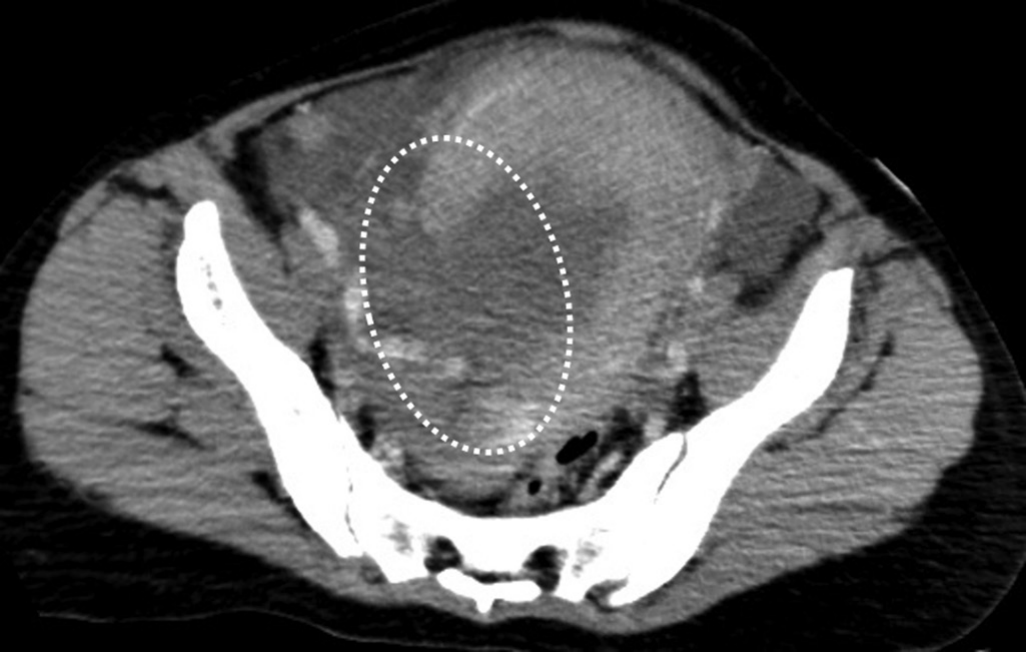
Contrast‐enhanced computed tomography axial image at the time of arrival. This image shows separation of the uterine wall and leakage of the contrast medium from the cervix to the right side of the uterine corpus.
